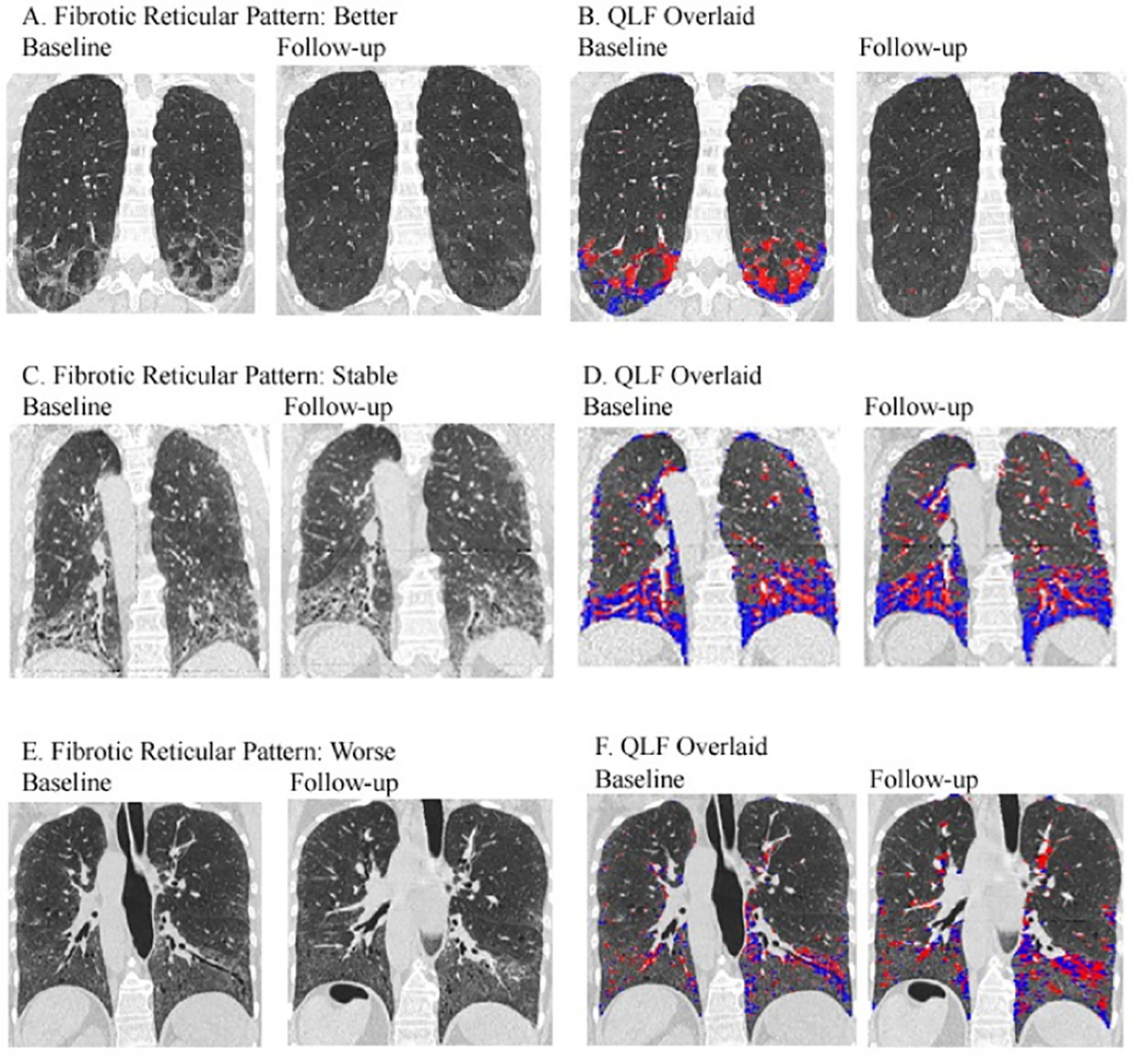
Representative pairs of baseline and follow-up HRCT images. (A) Better case: pulmonary fibrosis (PF) improved and ground glass opacity (GGO) worsened by the visual assessment in whole lung; (B) annotated HRCT of (A) with a CAD system: QLF and QILD in whole lung decreased, by 2.9% (3.9% to 1.0%) and 0.2% (19.9% to 19.7%), respectively. (C) Stable case; (D) annotated CAD of (C): QLF in the worst lobe (lower left) increased by 2% (64% to 66%). In whole lung, QLF and QILD increased, by 2.3% (39.5% to 41.8%) and 1.8% (72.4% to 74.2%), respectively. (E) Worse case: PF worsened and GGO improved; (F) annotated CAD of (E): QLF increased by 6.0% (28% to 34%) in the most severe lobe (lower right) and 1.8% (9.8% to 11.6%) in whole lung. QILD in whole lung was stable (41.3% to 40.5%).
